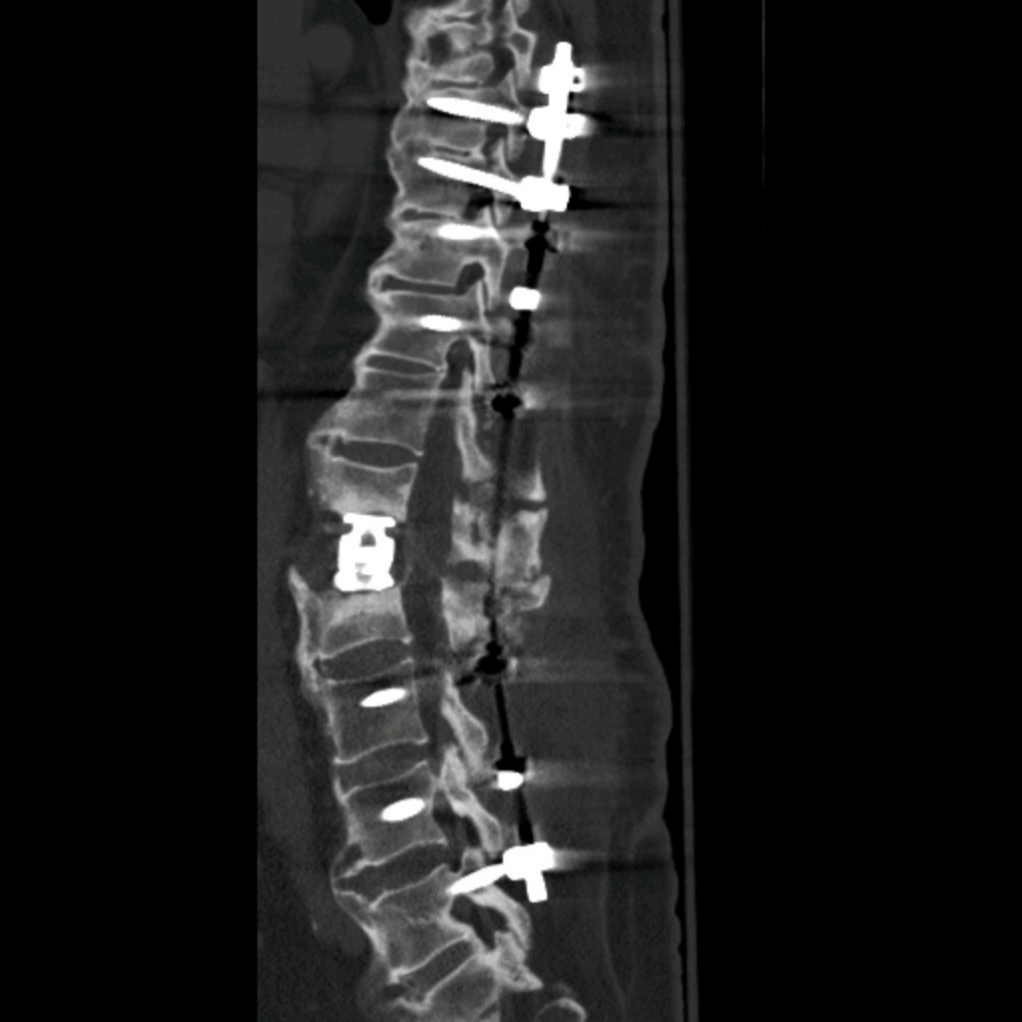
Sagittal postoperative CT This sagittal view of the postoperative CT of the thoracolumbar spine shows intact titanium rods and screws spanning from T8 to L5 with placement of an expandable titanium cage at L1-2.
